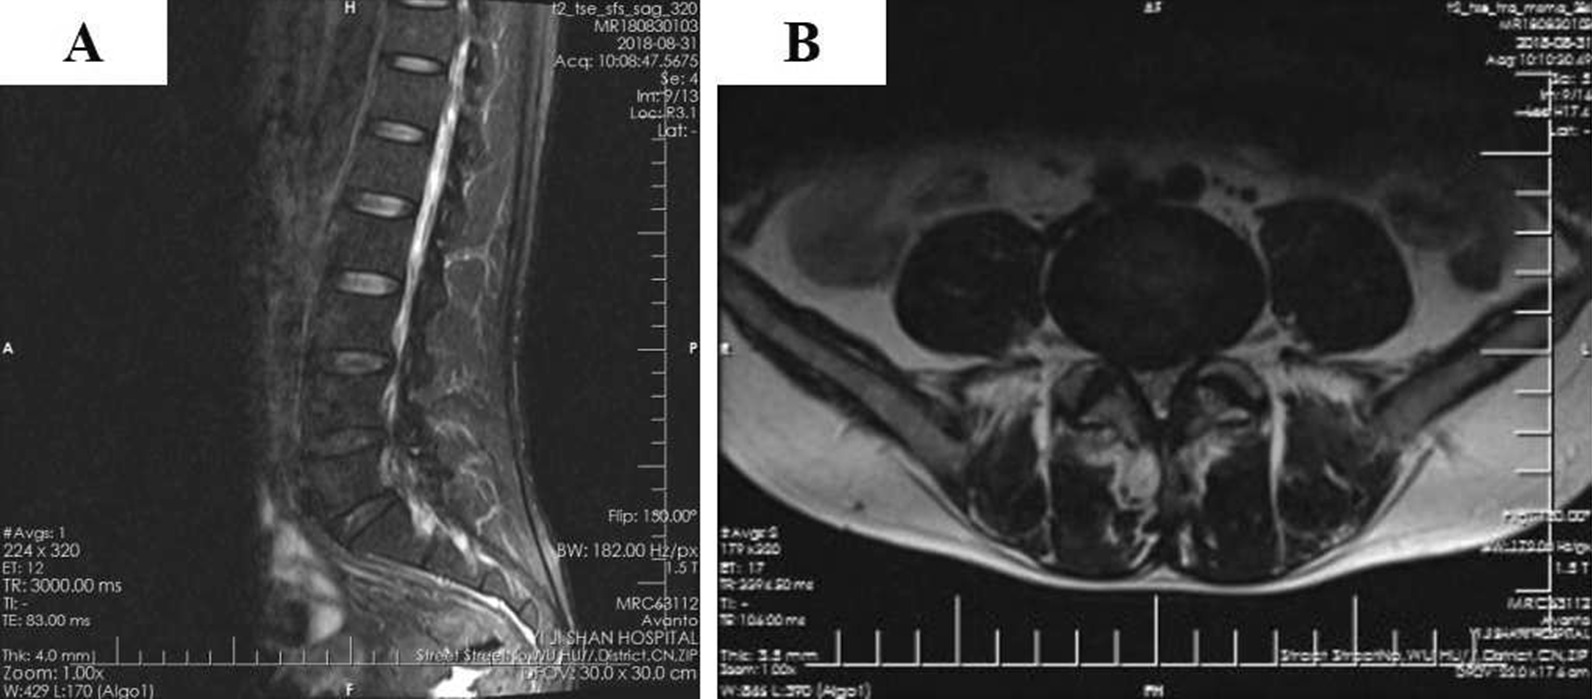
Sagittal (A) and transverse (B) fat-suppressed MRI of the lumbar spine indicating narrowing of the spinal canal at L4/5
